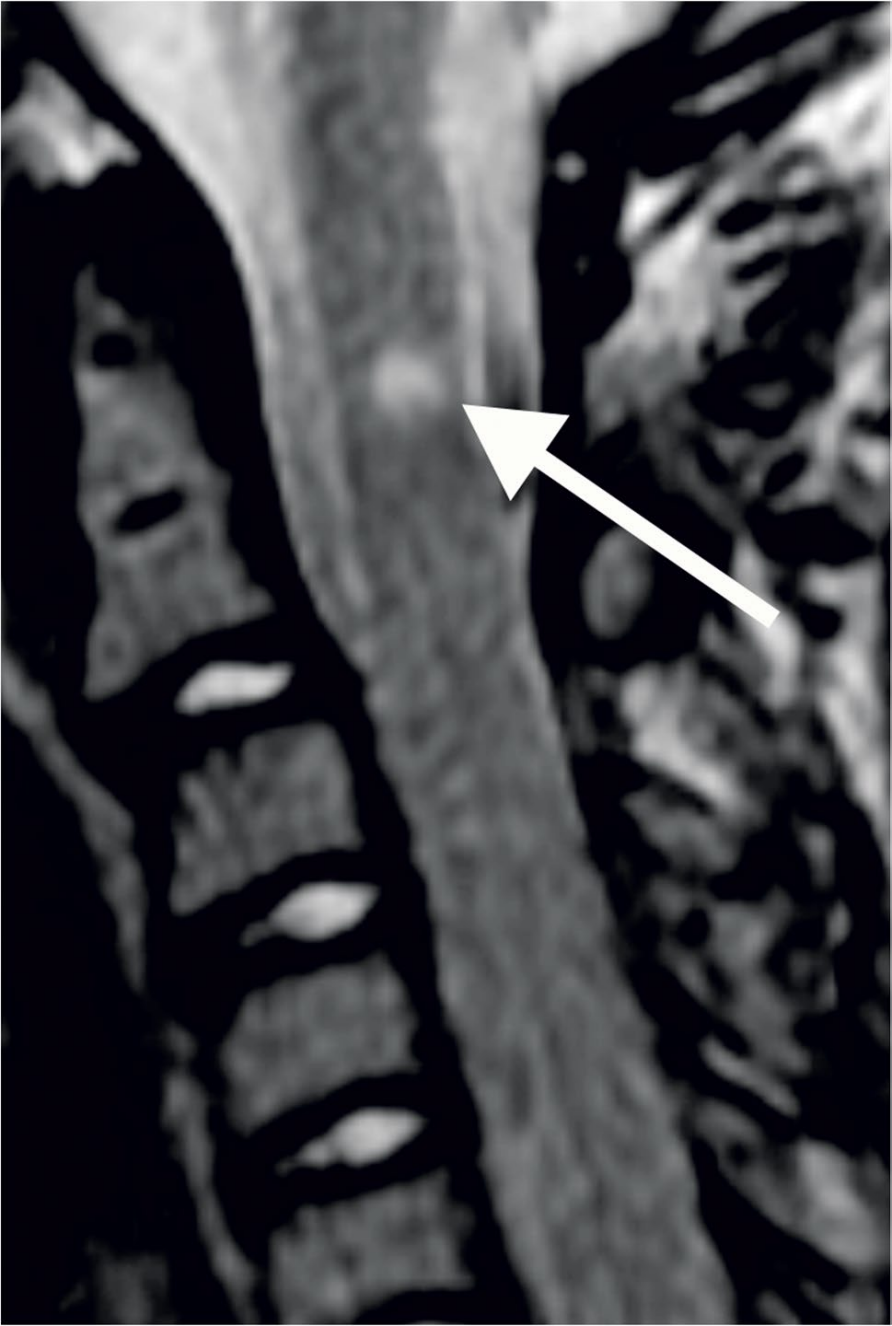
A sagittal T2 magnetic resonance image of the craniocervical junction in a 7-year-old girl with achondroplasia. T2 signal change is present in the cervical cord (*arrow*) without evidence of foramen magnum stenosis
